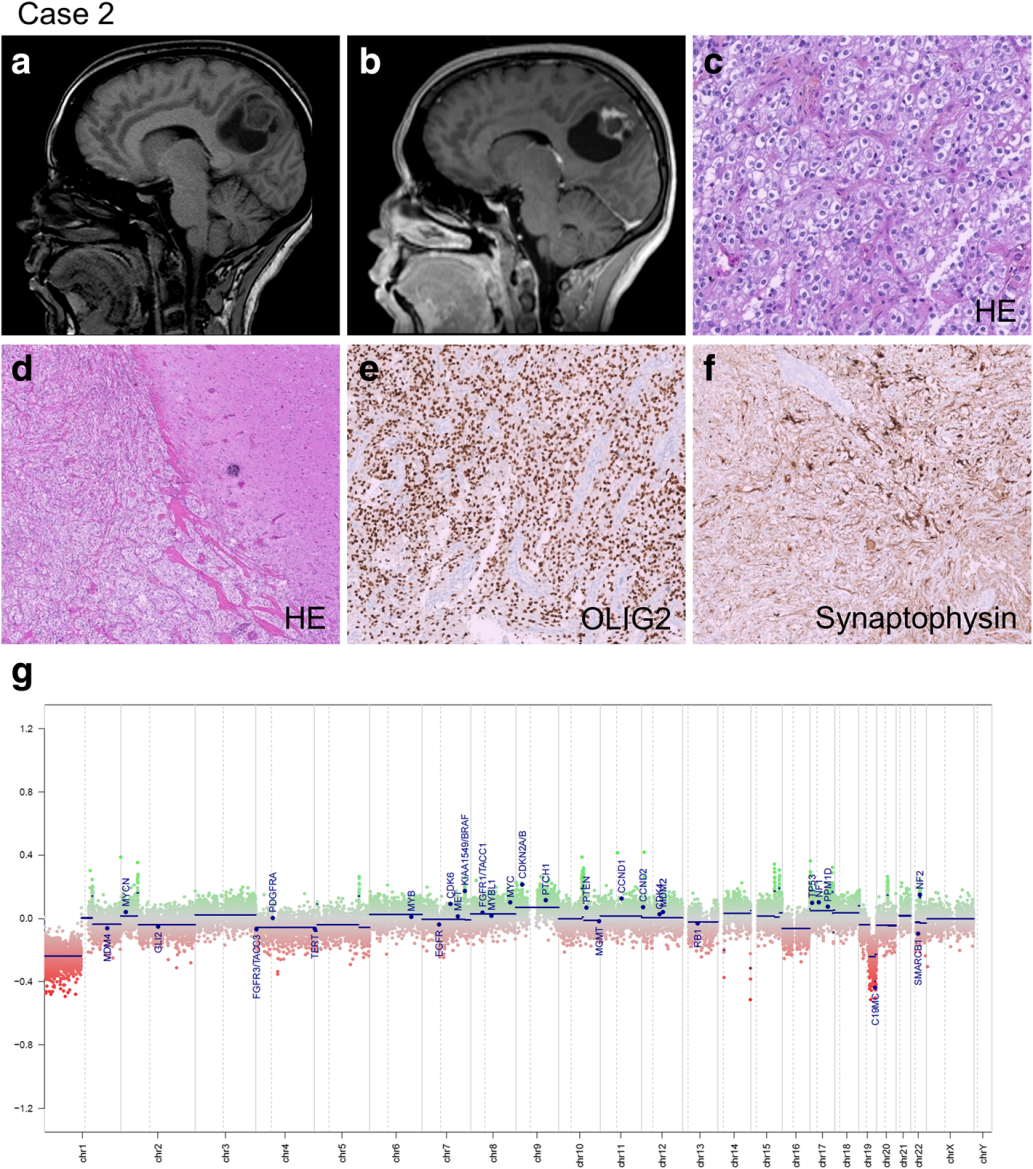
a, b MRI showed a cystic tumor with a mural nodule enhanced after gadolinium injection. c A proliferation of oligodendrocyte-like cells associated with a rich and branched vasculature. d Well demarcation of the tumor from the adjacent cortex. e, f The tumor cells strongly expressed OLIG2 and synaptophysin. g Copy-number profile derived from DNA methylation arrays revealed 1p/19q codeletion and *KIAA1549:BRAF* fusion
